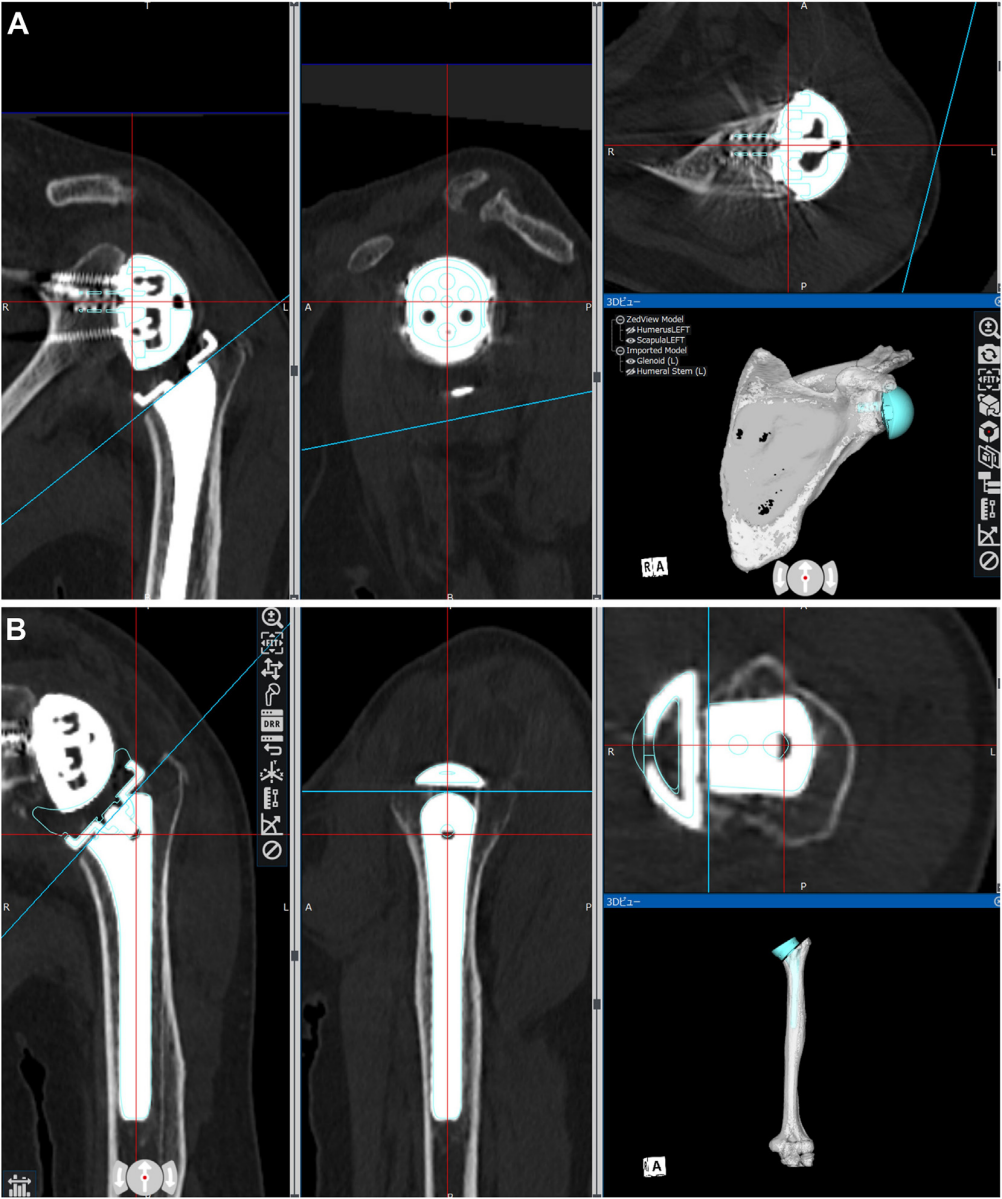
Method of matching implants. Computer-aided design (CAD) models of the Exactech Equinoxe Reverse Shoulder System for the implants in postoperative computed tomography data. A: Matching with the scapular component. B: Matching with the humeral component.
